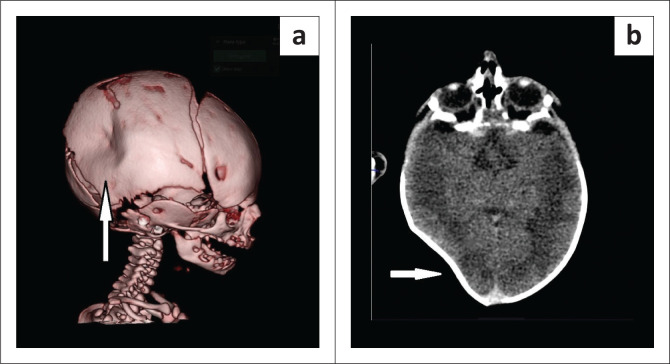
(a) Three-dimensional volume-rendered CT reconstruction demonstrates a focal depressed deformity (white arrow) of the right parieto-temporal skull without cortical discontinuity, and (b) axial CT image (soft tissue window) shows the corresponding inward depression (white arrow) of the calvarium.
